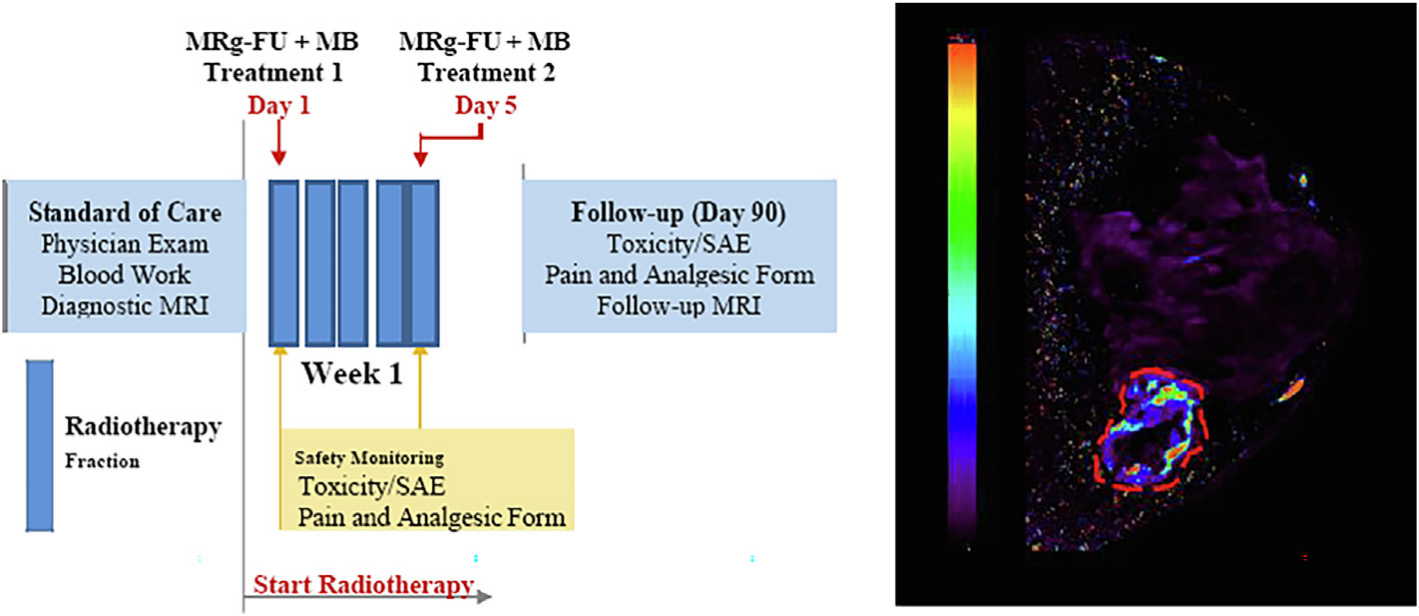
(Left) Treatment outlines. Fractionation regimens: Two (2) MRg-FUS + MB treatments are scheduled as indicated. There will be two permitted stratifications for radiation dose: 2000 cGy/5 fractions. (Right) Preliminary DCE MRI K-Trans Data. The dashed line indicates a tumor. The color bar indicates relative DEC k-trans parameter data From [84].
